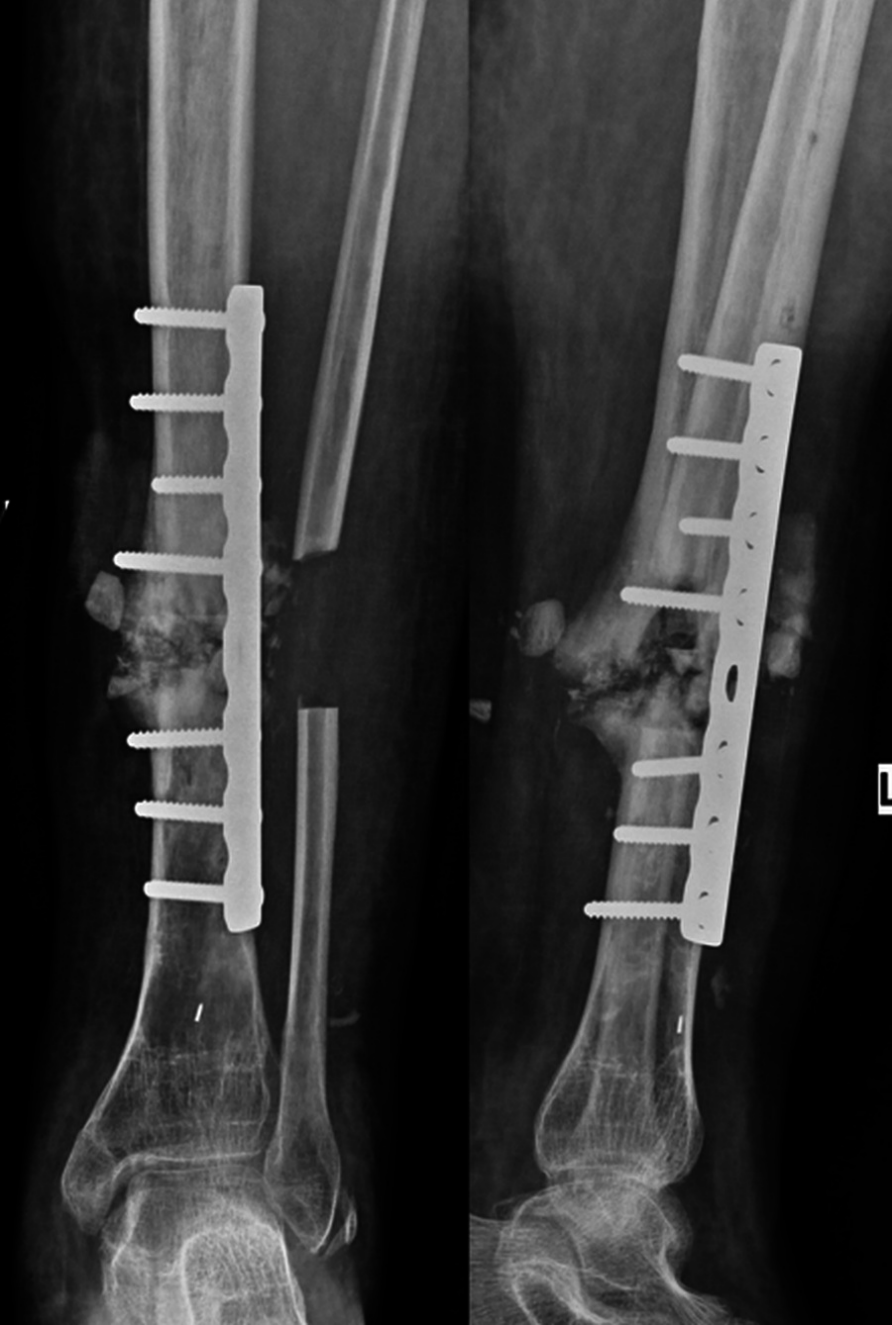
Postoperative radiographs showing lateral plating of the tibia, fibulectomy, and bone grafting.
